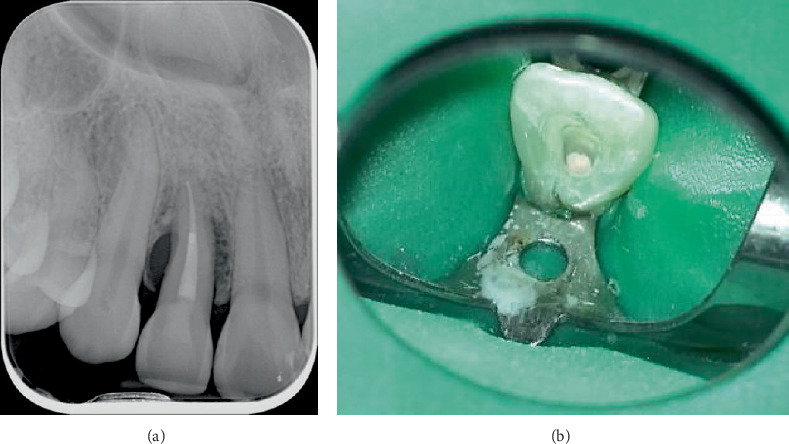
Obturation of Tooth 12. (a) Periapical radiograph. (b) Intraoral photograph.
